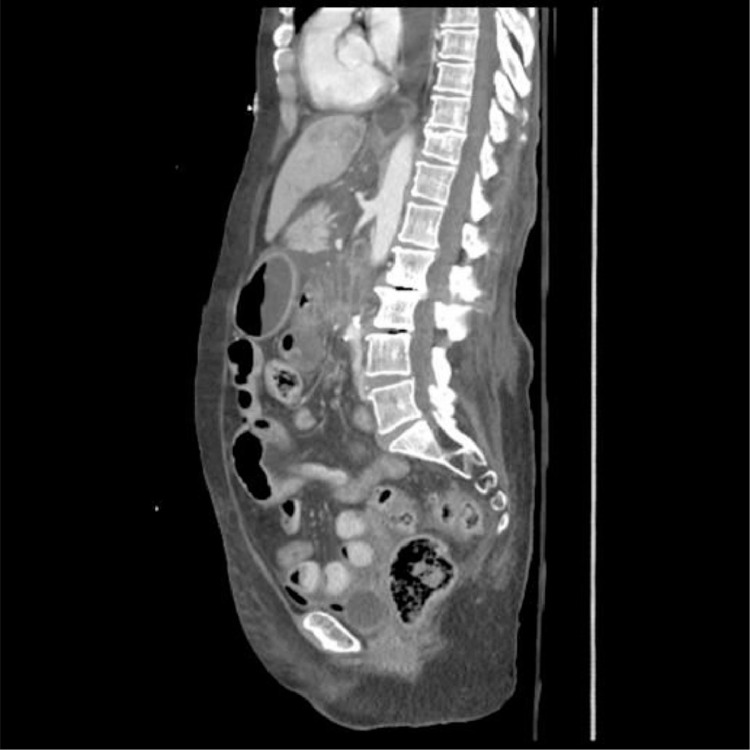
Sagittal image of contrast-enhanced abdominal CT, showing the common origin of the celiac and superior mesenteric artery.
